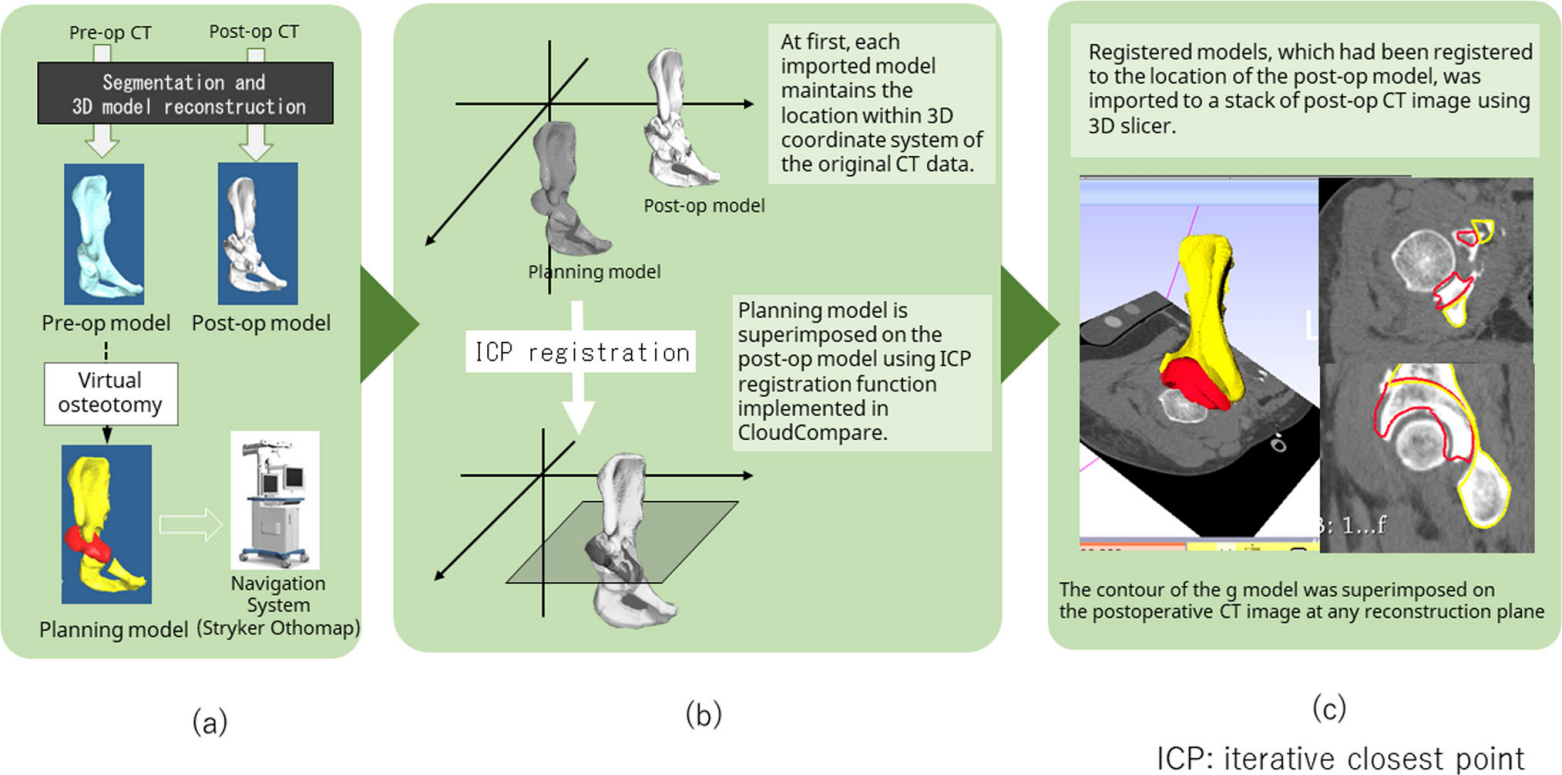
Use of the model-based registration method to compare the preoperative planned and postoperative models. a A three-dimensional (3D) model of the pelvis and proximal femur is created based on the computed tomography (CT) images obtained before and after surgery. b First, each imported model maintains the location within the 3D coordinate system of the original CT data. The antero-pelvic plane was represented as an *X*–*Y* plane, and the line passing through the bilateral center of the femoral heads was denoted as the *X*-axis, while the axis vertical to *X*–*Y* plane passing through the femoral head of operative side was denoted as the *Z*-axis. The planned model is superimposed over the postoperative model using the iterative closest point (ICP) registration function implemented in CloudCompare. c Registered models, which had been registered to the location of the postoperative model, were imported to a stack of postoperative CT images using 3D Slicer; this software enables the display of the cross section of the pelvis in any plane
